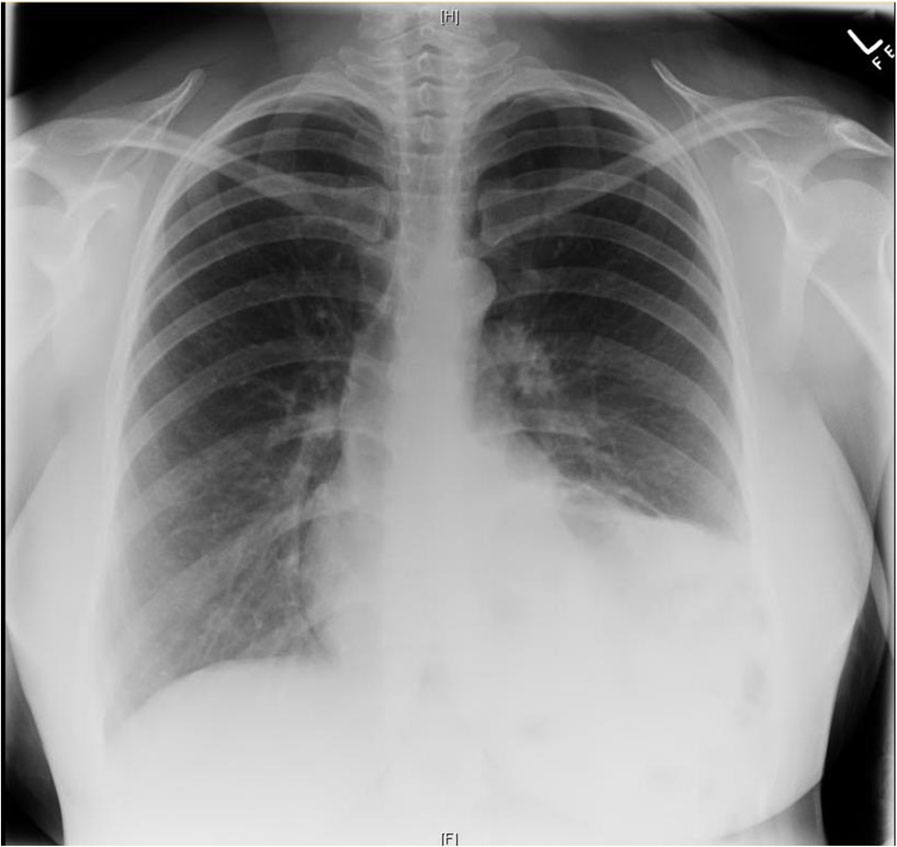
Chest Xray from first presentation at 13 weeks showing left lower lobe opacification. While initially thought to be left lower lobe pneumonia this is likely to represent the diaphragmatic hernia even at this early gestation
